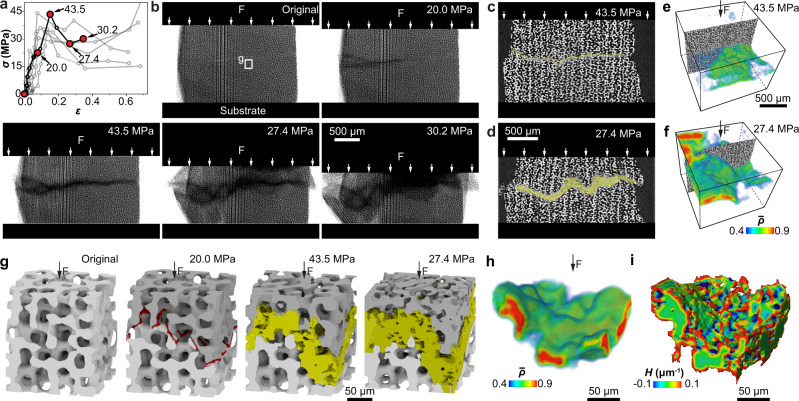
Synchrotron X-ray µ-CT based in situ mechanical analysis. a The stress–strain curves of stereom from 11 in situ tests. b X-ray projection images of deformation stages corresponding to the curve with highlighted data points in (a). c, d Vertical reconstruction slices and e, f corresponding 3D renderings of relative density ($$\bar{\rho }$$), showing the evolution of damage bands in stereom (shaded in yellow in c, d) at applied stress *σ* = 43.5 MPa and 27.4 MPa, respectively. c, d Share the same scale bar. e, f share the same scale bar and color bar. The color in e, f is set transparent when $$\bar{\rho } \, < \, 0.4.$$
